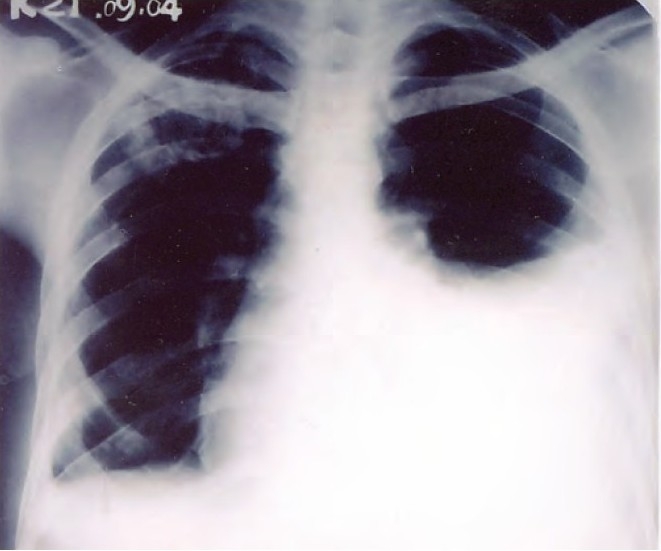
Xray chest PA view showing heterogeneous opacity in the right upper zone with blunting of the right costophrenic angle and moderate pleural effusion on the left side.
